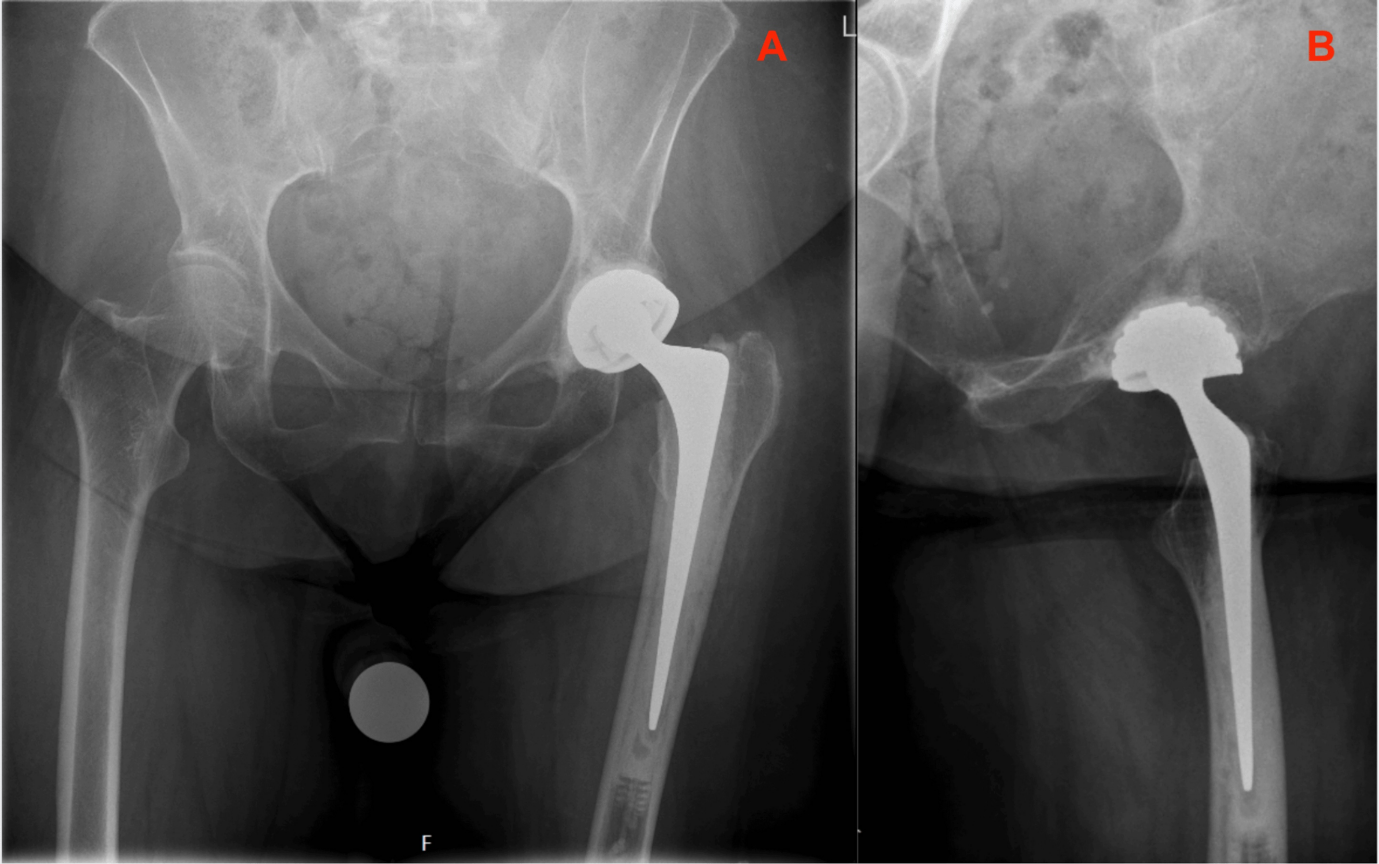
Primary Total Hip Arthroplasty, six weeks postoperative Six weeks postoperative radiographs of the primary THA with CPT femoral stem, performed for a femoral neck fracture. 2A: Anterior Posterior view of the Pelvis; 2B: Lateral view of the Left Hip THA: Total Hip Arthroplasty; CPT: Collarless, Polished, Double-Taper
